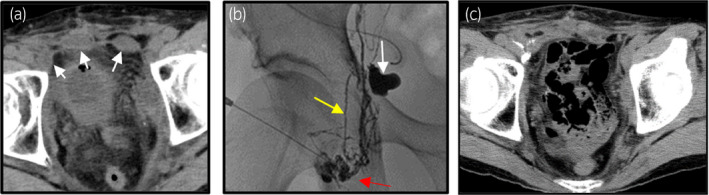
Representative images of Case 2 during treatment. Abdominal CT shows three sites of small lymphocele in front of the bladder (a: axial image, white arrows indicate the small lymphoceles). Representative lymphangiography image of Case 2. The right side of the lymphangiography shows lymphatic leakage at the upper stream of the right lymphatic vessel (b: red arrow indicates the right inguinal node; yellow arrow indicates the right lymphatic vessel; and white arrow indicates the lymphatic leakage site.). Abdominal CT taken 18 days after RRP (c: axial image). The lymphoceles have almost completely disappeared.
